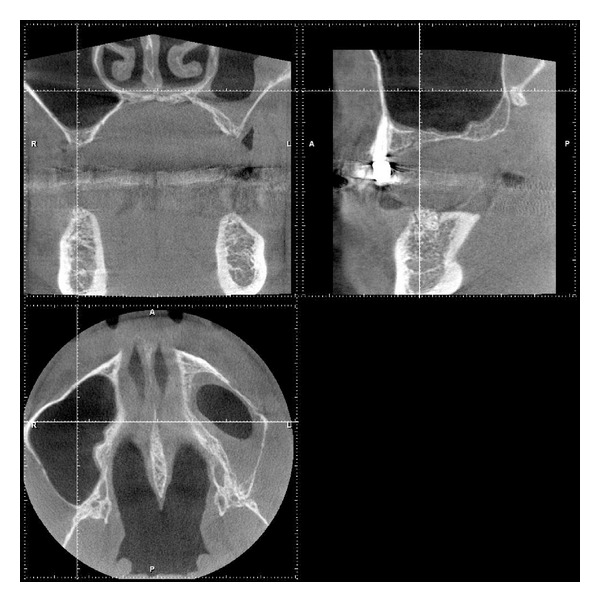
Case 2: presurgical situation in transversal, paramedian sagittal and horizontal CBCT-scan.
